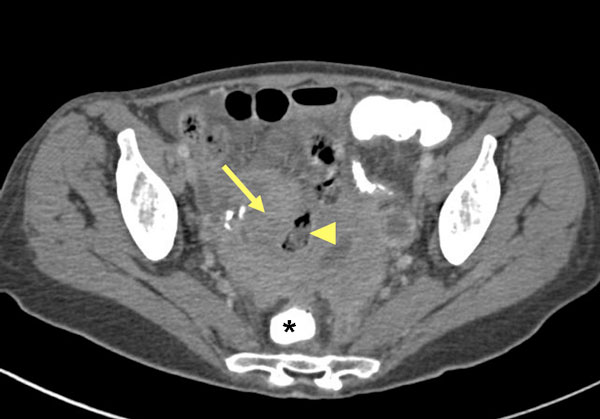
Computed tomography of the pelvis. A large conglomerate tumor *(arrow)* with hypodense and isodense fractions that surrounds and partly compresses intestinal loops of the ileum *(arrowhead)* is shown. There is no sharp demarcation of the lesion to the rectum that is filled with contrast agent *(asterisk)*.
